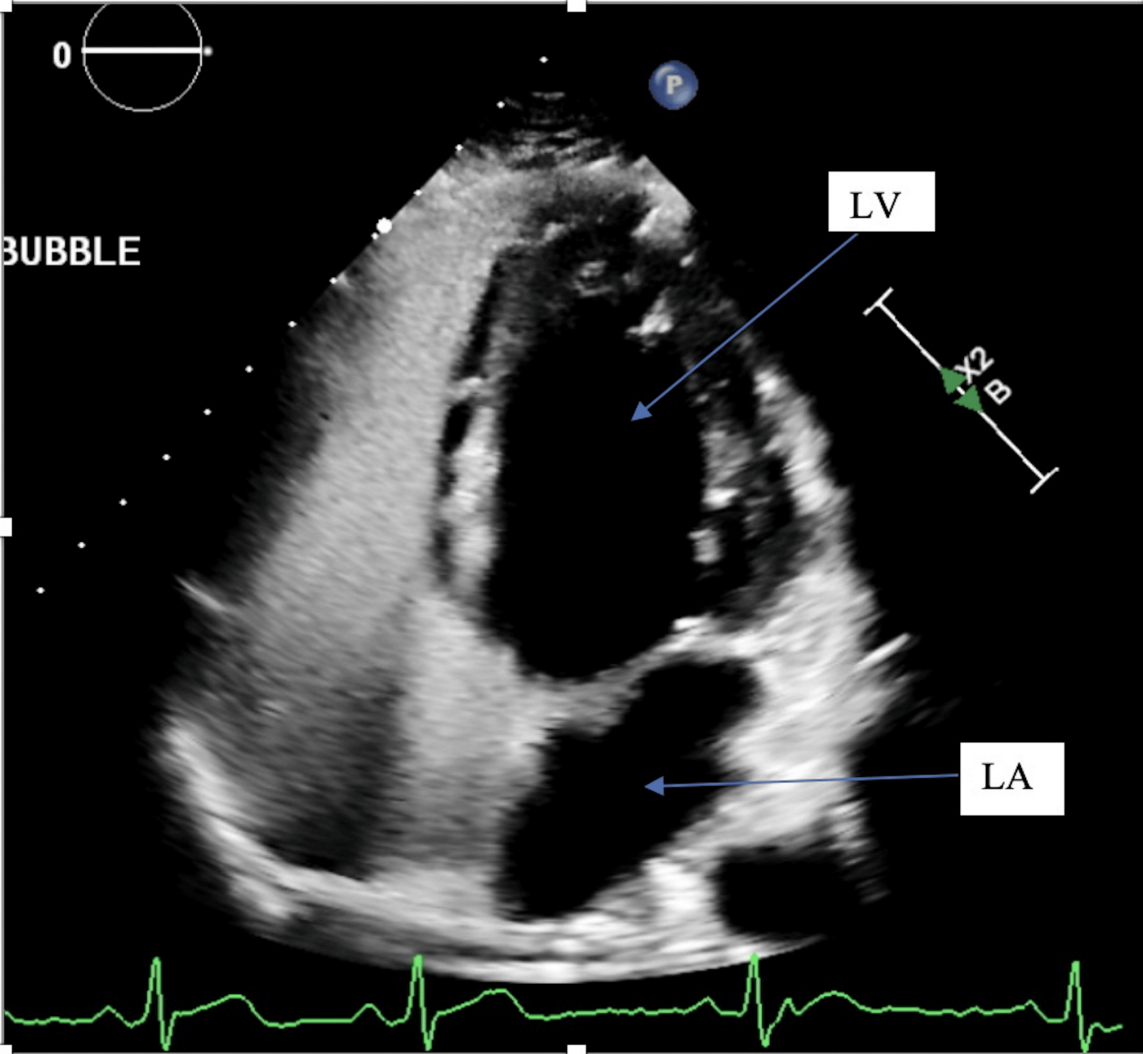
Echocardiogram showing no evidence of bubble in the left ventricle (LV) or left atrium (LA) within the six cardiac cycles
